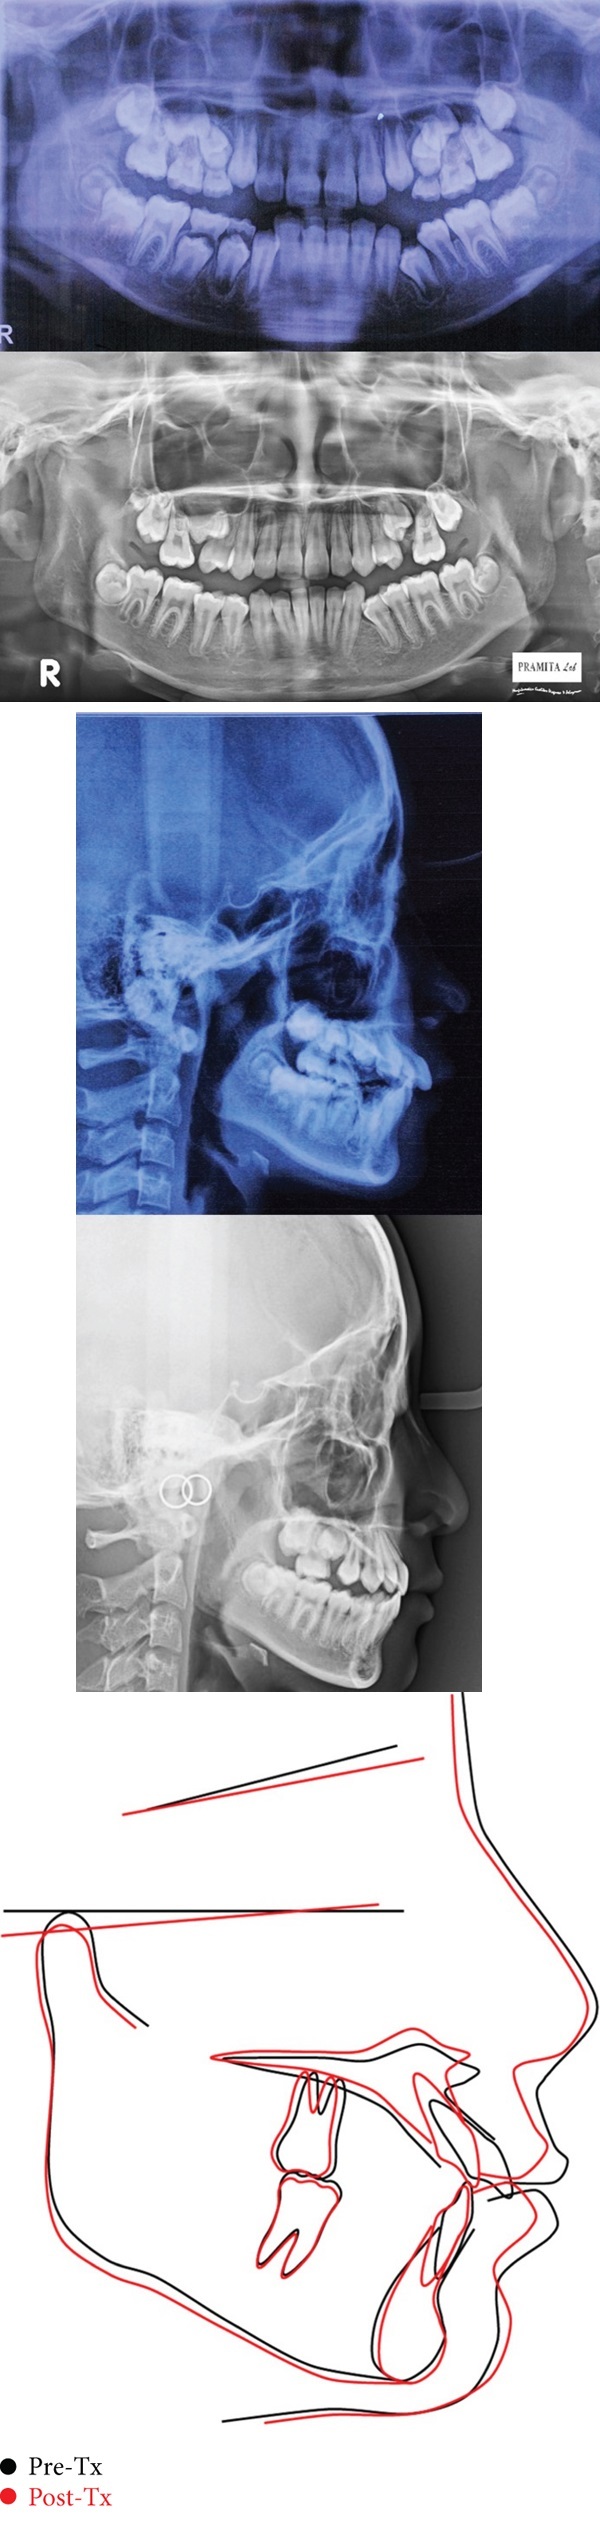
(a) Pre- and post-treatment panoramic radiographs, (b) lateral cephalometric, and (c) tracings of lateral cephalometric superimposed; black lines represent tracing before treatments, and red lines show the tracing after treatments.
